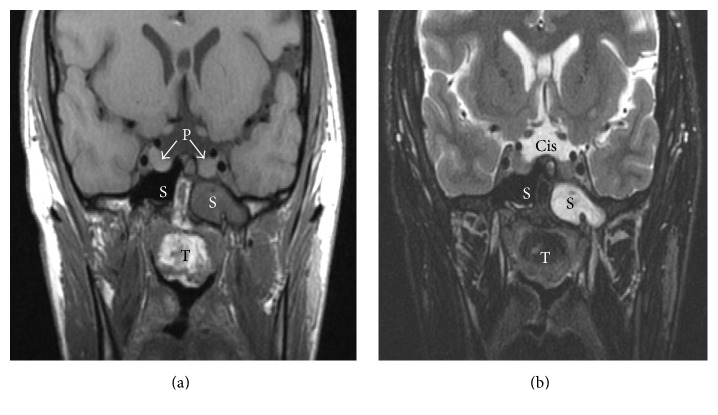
Magnetic resonance imaging (MRI) of pedunculated skull base lesion. (a) Coronal T1 MRI clearly demonstrates the tumor extending from intracranial midline defect into the nasopharynx. The bright intensity signal within the tumor (T) and stalk between the sphenoid sinuses (S) on T1 sequence represents fat, nearly pathognomonic of teratoma. Pituitary duplication (P with arrows) is best appreciated on T1 imaging as well. Of note, the left sphenoid sinus was noted to have mucosal thickening at the time of MRI compared to CT imaging (Figure 2). (b) Coronal T2 sequence at same slice location highlights the enlarged midline basilar cistern (Cis) with hyperintense signal representing cerebrospinal fluid.
